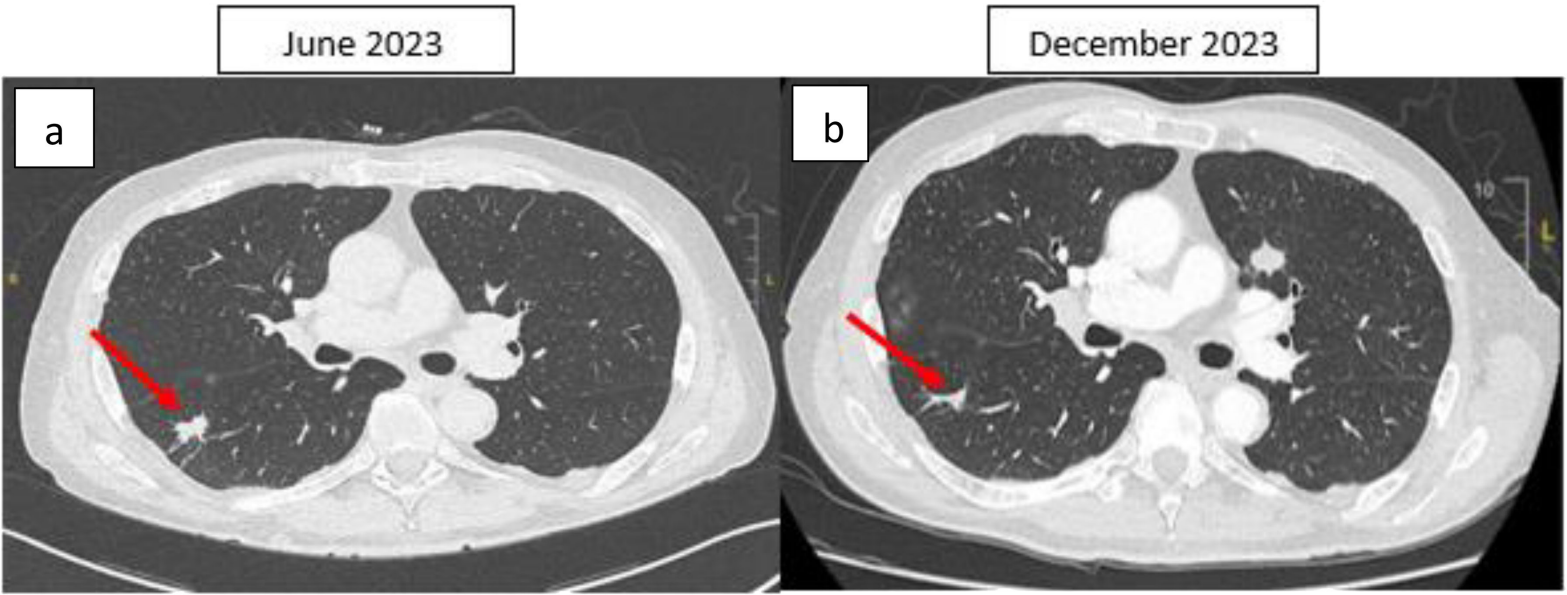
Thoracic CT scan, axial section: (A) Spiculated nodule in the apical segment of the right lower lobe initially measuring 20 × 11 mm, with some spicules reaching the fissural and parietal pleura. (B) After four months of ICI therapy, the nodule was reduced to 15 × 3 mm. The right lung lesion demonstrates a reduction in size between the June 2023 and December 2023 CT scans. However, a growing nodule is observed in the left lung on the December 2023 scan. This may be explained by the fact that the June 2023 scan was performed prior to the initiation of immunotherapy (which began in August 2023). As a result, the left lung nodule (HCC metastases) could have continued to progress during the interval between the scans, before the therapeutic effects of immunotherapy were fully realized. This temporal difference in treatment response may account for the observed discrepancies in lesion size. The red arrow indicates the lung tumor.
